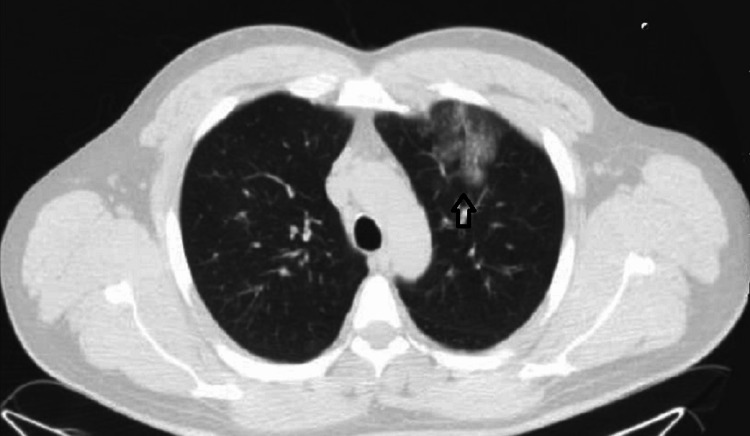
Chest computed tomography scan on previous admission Chest computed tomography scan showing pulmonary infiltrate during a previous admission because of severe asthma exacerbation.
